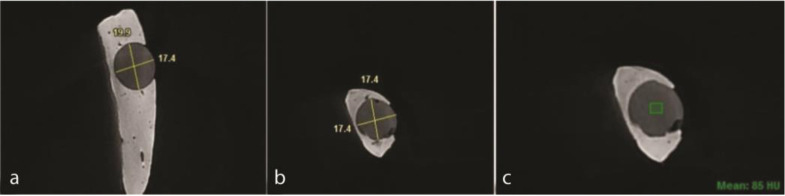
Measurement of lesion sizes, a: Axial, b: Coronal CBCT images
